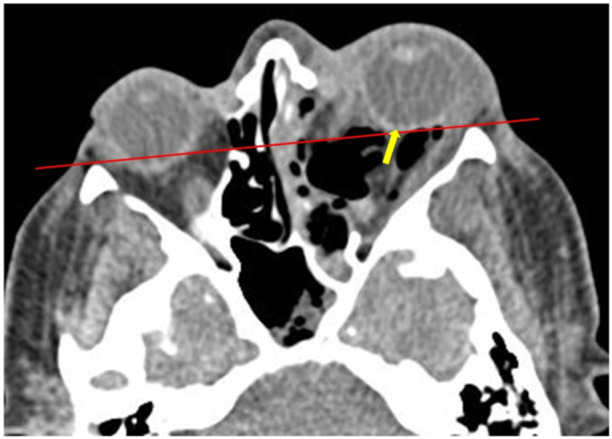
Axial noncontrast CT image in soft tissue window demonstrating left orbital involvement with proptosis. The arrow indicates anterior displacement of the globe beyond the interzygomatic line (red line).
